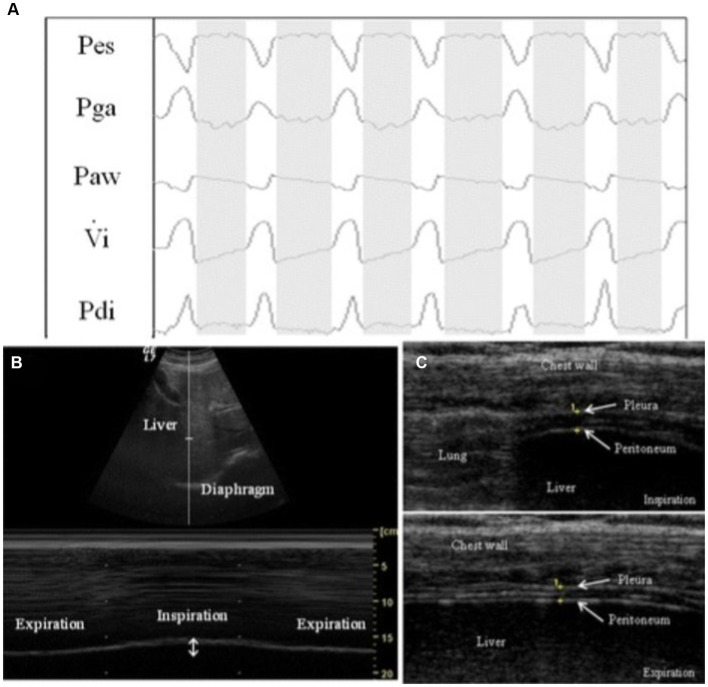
Example of respiratory tracings with ultrasound measurements during a pressure support of 0 cm H_2_O (PS_0_ step). (A) Respiratory tracings showing: Pes, esophageal pressure; Pga, gastric pressure; Paw, airway pressure; Vi, respiratory flow; Pdi, transdiaphragmatic pressure. The white columns represent inspiration and gray columns represent expiration phases. (B) Ultrasound view of diaphragm excursion during spontaneous breathing in B-mode (upper) and M-mode (lower). (C) Ultrasound view of the diaphragm in the zone of apposition during inspiration (upper) and during expiration (lower). The diaphragm is the three-layer structure in the middle consisting of a hypoechoic central layer sounded by an echogenic diaphragmatic pleurae and peritoneum, as indicated by the yellow arrows. This image was adopted with the permission of the Creative Commons license. No changes were made to this image. The Creative Commons Public Domain Dedication waiver (http://creativecommons.org/publicdomain/zero/1.0/) applies to this image (40).
